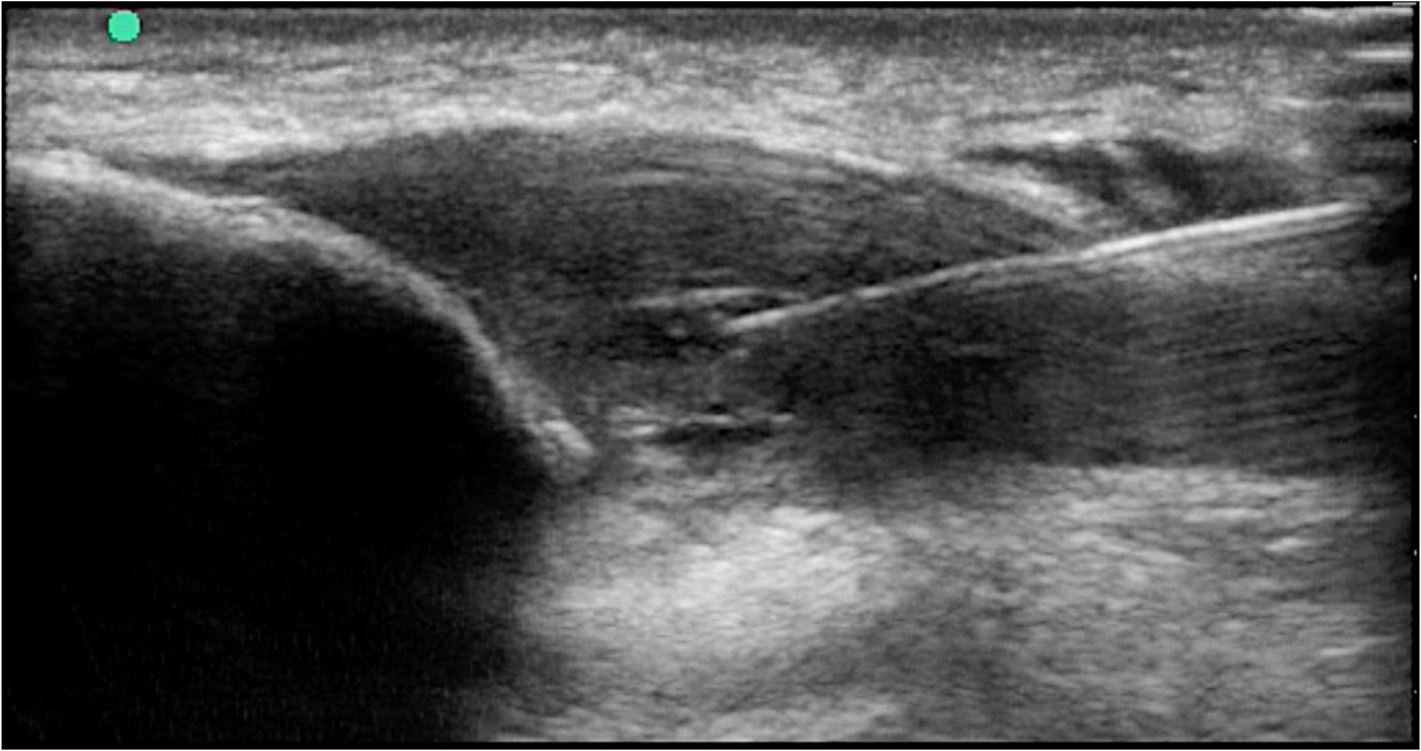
Ultrasound guided cell injection into the hypoechoic area of the proximal patellar tendon
